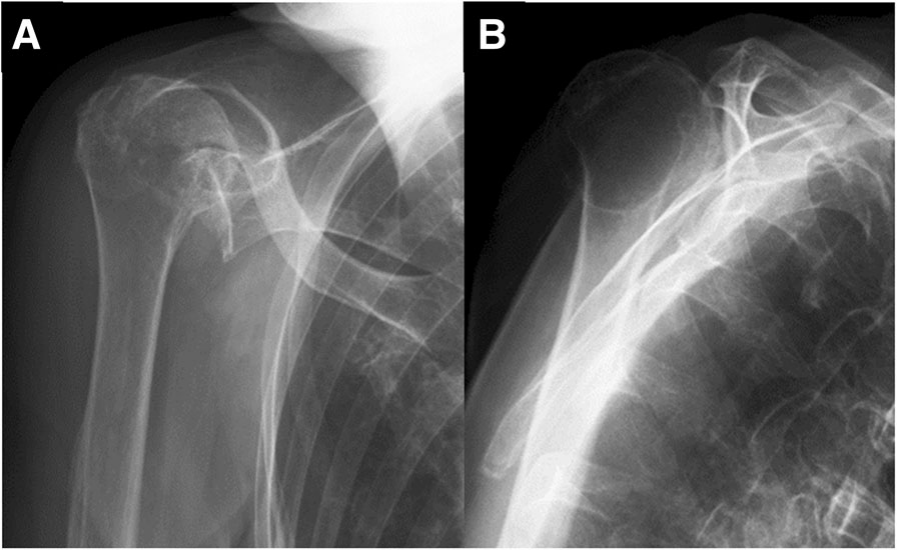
X-ray radiography images demonstrating the right humeral head dislocated upward in a direction posterior to the acromion (a, b)
